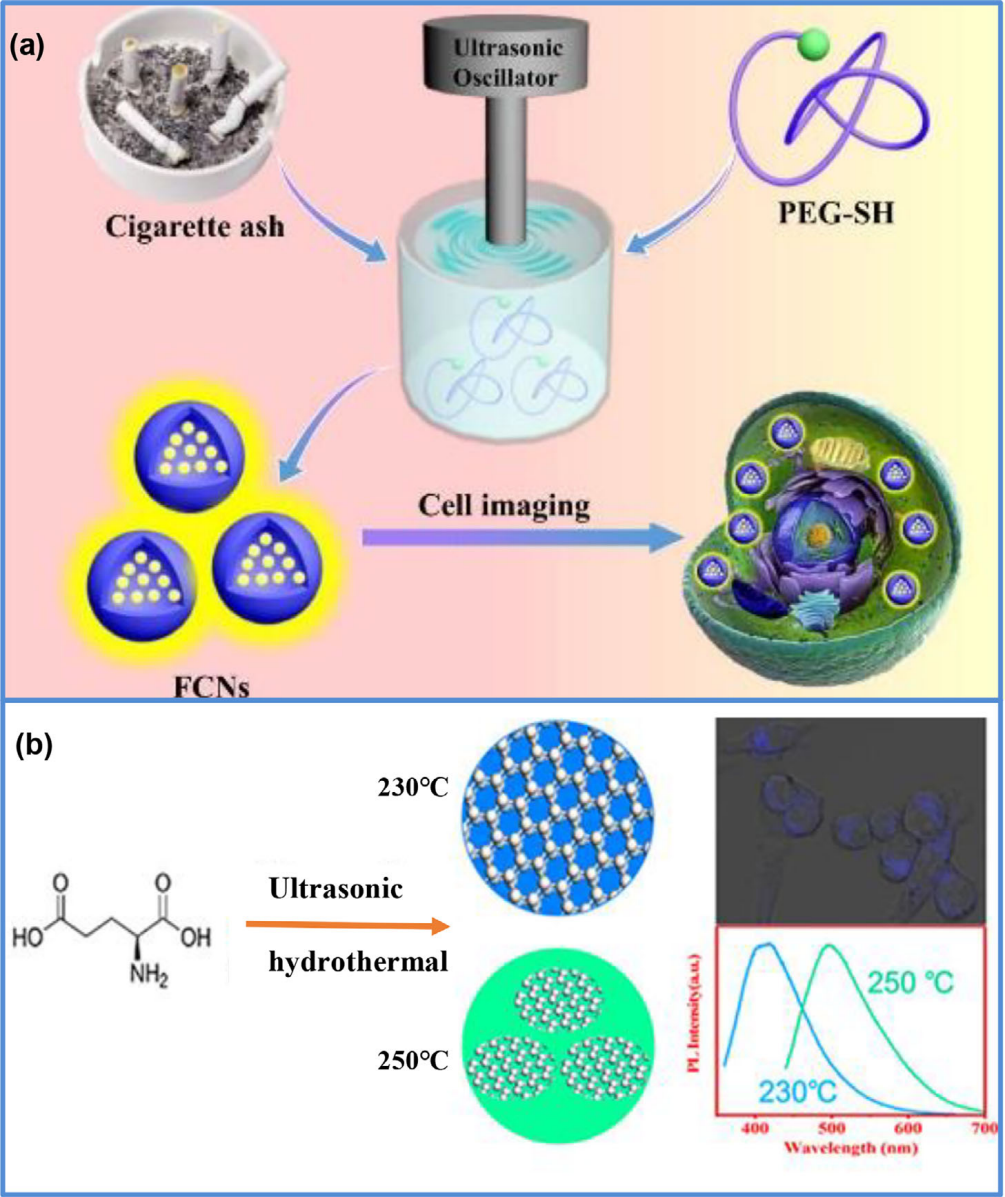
(a) CQDs were synthesized by ultrasonic method [87, 88]. Reproduced with permission of Ref. Copyright 2018, Elsevier. (b) LCQDs were prepared by microwave assisted hydrothermal method [85]. Reproduced with permission of Ref. Copyright 2021, American Chemical Society.
